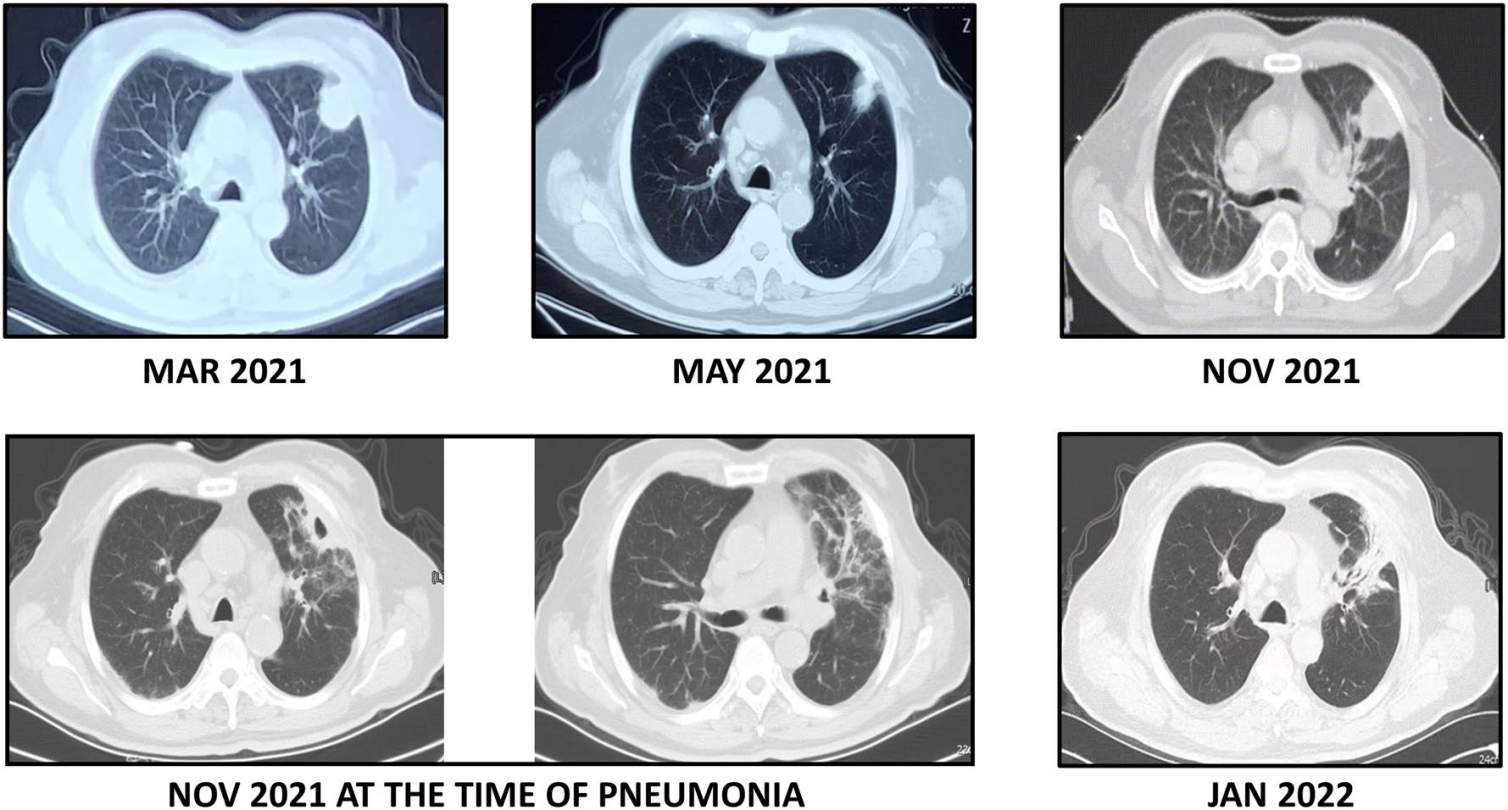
Chest CT scans of the patient with lung squamous cell carcinoma (LSCC) at different time points during treatment
